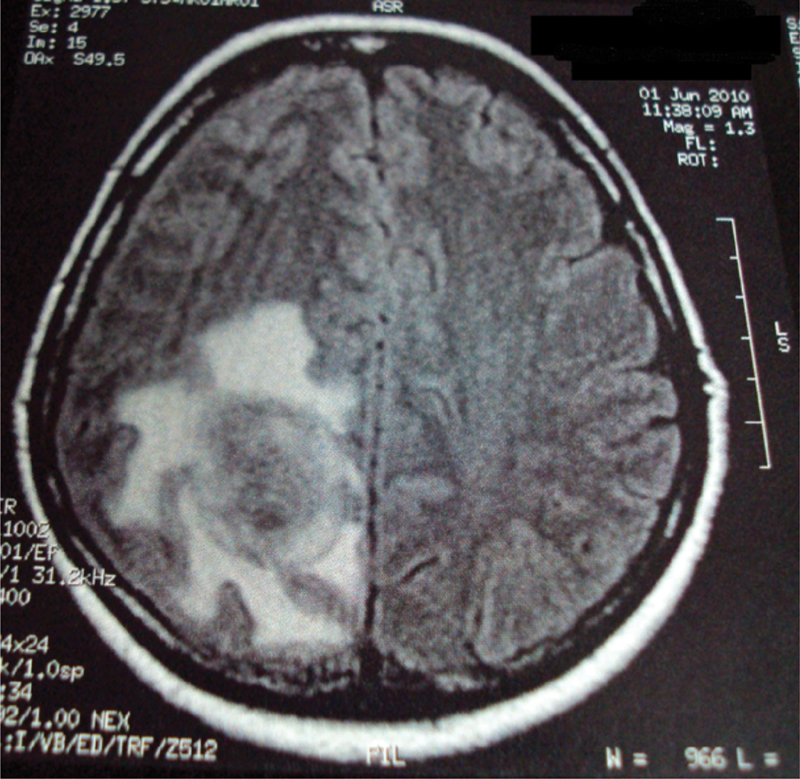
Magnetic resonance imaging showing an expansile mass in the right parietal lobe consistent with hematogenous implant.
